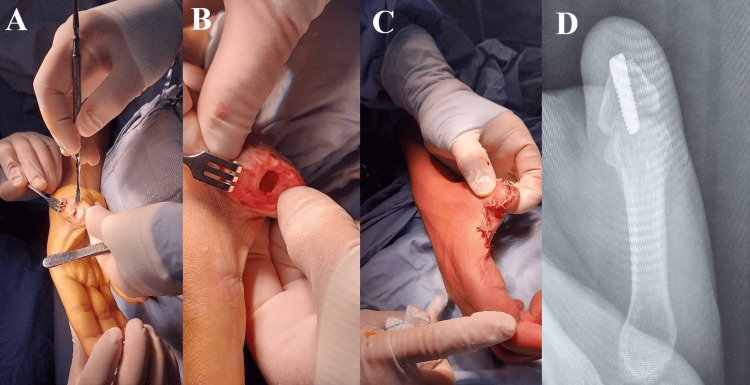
Intraoperative images (A, B) Intraoperative images demonstrating the first stage, including preparation of the bone for insertion of a dental implant. (C) Widening of the first web space to improve prosthesis fitting and thumb grip. (D) Radiographic image showing the dental implant in position.
